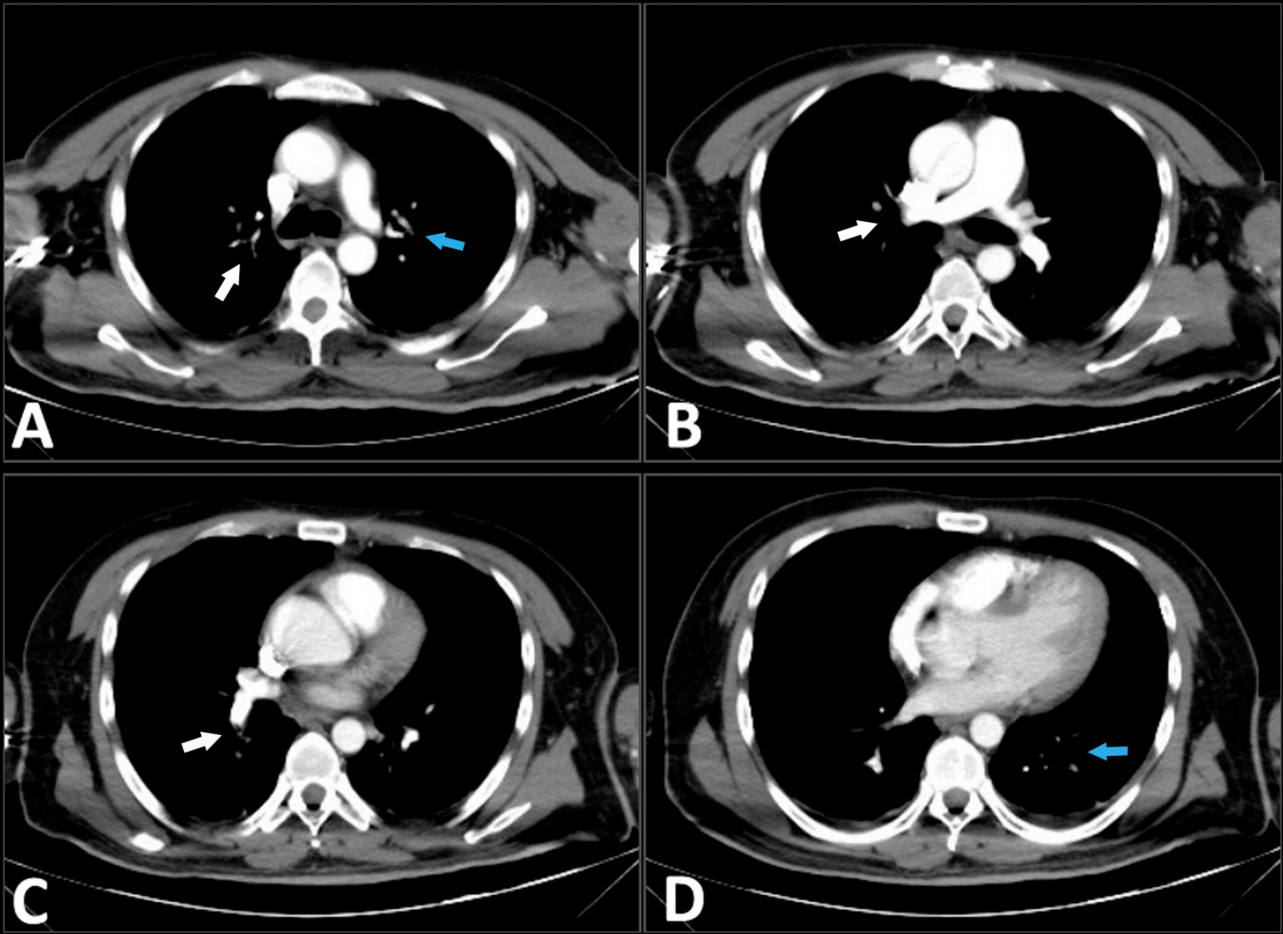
Contrast-enhanced CT pulmonary angiogram Filling defects (white arrows) are observed in the right lobar pulmonary artery and distal segmental arteries (A, B, and C). In the left hemithorax, filling defects (blue arrows) are observed in apical segmental arteries, as well as in some lower segmental arteries (A and D). CT: computed tomography
